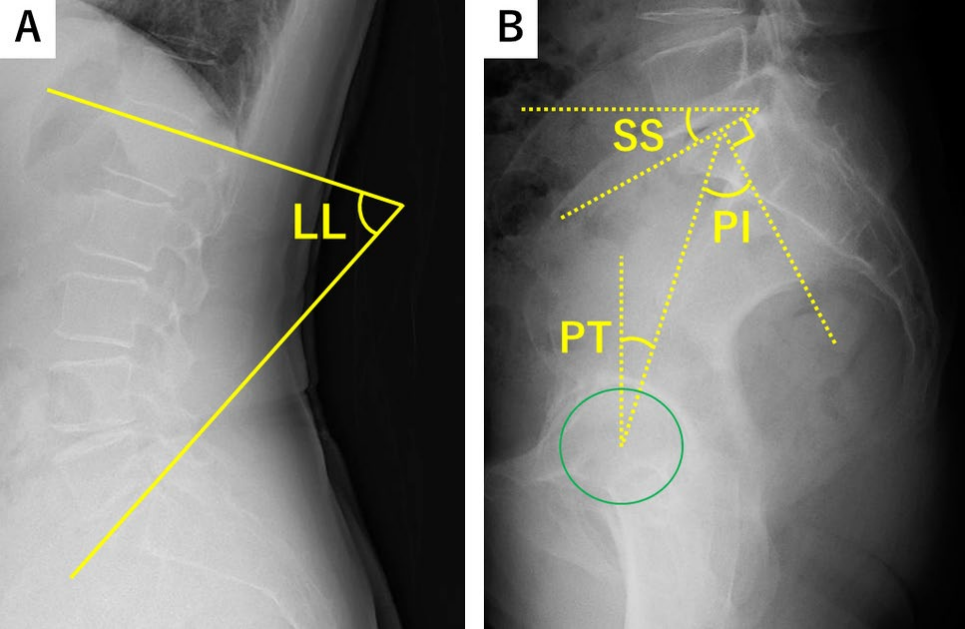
Methods of radiographic measurement of spinopelvic parameters. Methods for lumbar lordosis (LL), sacral slope (SS), pelvic tilt (PT), and pelvic incidence (PI) have been described. All the measurements were obtained using standing lateral plain radiographs. *LL* lumbar lordosis, *SS* sacral slope, *PT* pelvic tilt, *PI* pelvic incidence
